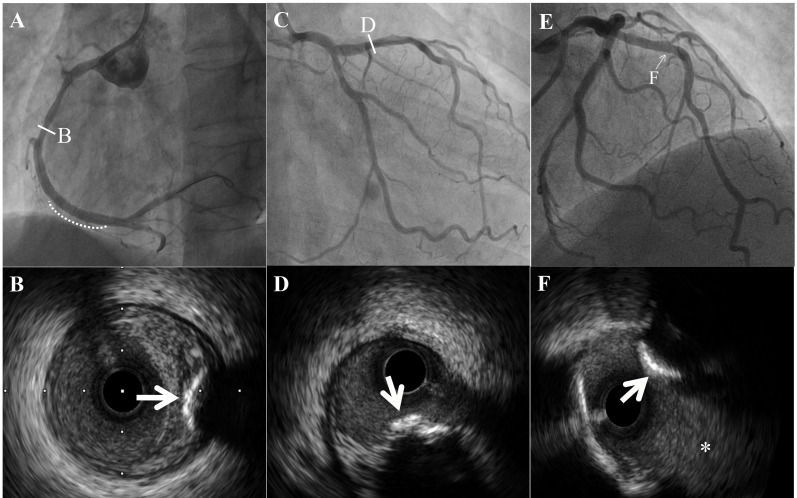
Intravascular ultrasound images of a calcified nodule (CN). (A)
